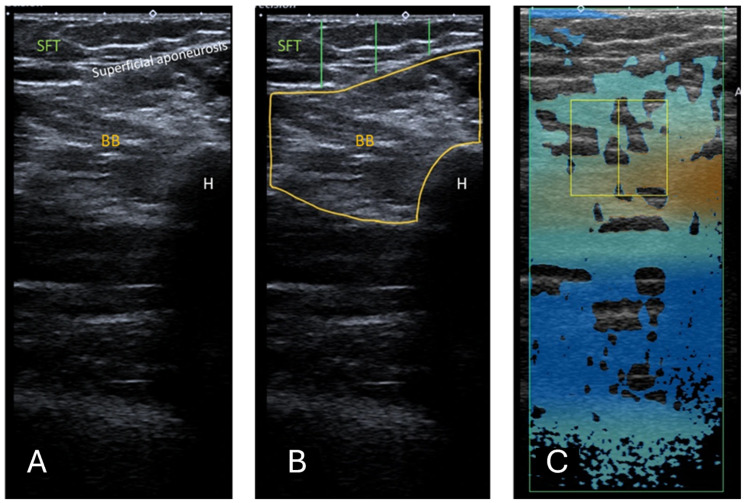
(A) Ultrasound B-mode image of the BB. The distance between the epidermis and superficial aponeurosis is defined as SFT. (B) Segmented image of the BB, including the muscle cross-sectional area and the lines marking the thickness of the SFT from the lateral, central, and medial aspects of the BB. The measurement of the SFT was taken at 25%, 50%, and 75% of the length of the total muscle visible on ultrasound, respectively. (C) Attenuation imaging of the BB. The green box indicates the area of interest (AOI), and the yellow box indicates the region of interest (ROI) placed within the BB. BB = biceps brachii, SFT = subcutaneous fat tissue, H = humerus.
